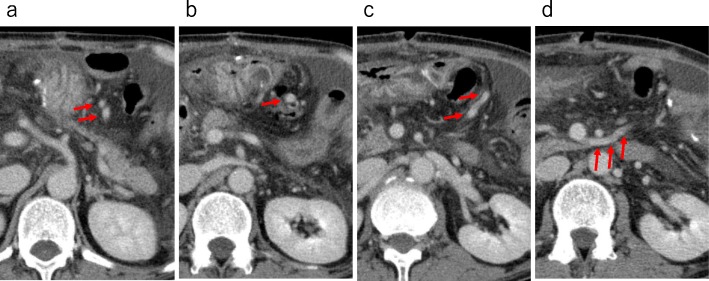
Contrast-enhanced CT scan. a–d Dilatation the branches of jejunal vein of the ascending jejunal limb (arrow)
